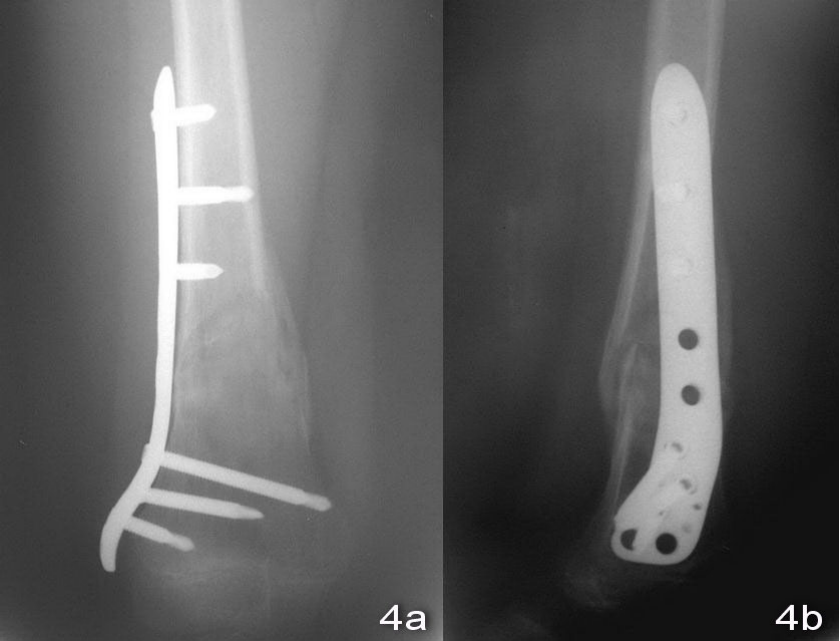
X-ray of right knee showed united fracture.
